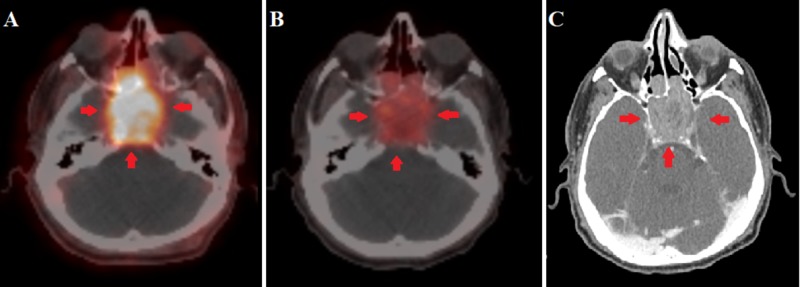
Gallium PET/CT scan (A, B); CT scan with contrast (C) (A) Baseline gallium positron emission tomography/computed tomography (PET/CT) scan showing the large hypermetabolic sphenoid sinus tumor; (B) PET-CT scan three months after intensity-modulated radiation therapy (IMRT) showing a significant decrease in metabolic activity; (C) CT scan at the last follow-up three years after diagnosis showing stable disease.
